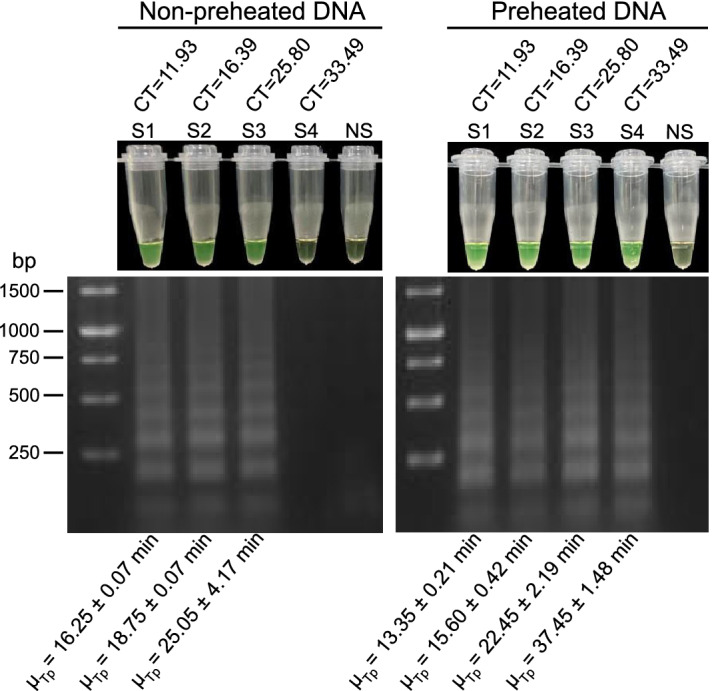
Evaluation of the uvrD_2-based LAMP assay using preheated and nonpreheated template DNA from a clinical sample panel with a bacterial load gradient. Preheated DNA consisted of denaturing template DNA at 96 °C for 5 min prior to loading of the sample for the LAMP reaction. Reactions were considered positive for LAMP products if they had both a color change from brown to green and a ladder-like banding pattern on agarose gel after electrophoresis. S1–S4, clinical samples positive for *B. pertussis* DNA with different cycle threshold (CT) values obtained by multitarget qPCR; NS, clinical samples negative for *B. pertussis* DNA; bp, base pair; µ_Tp_, mean time to positivity (Tp) in minutes (min) in the LAMP reaction (mean ± SD). Original gels are presented in online Supplementary Fig. S2.
